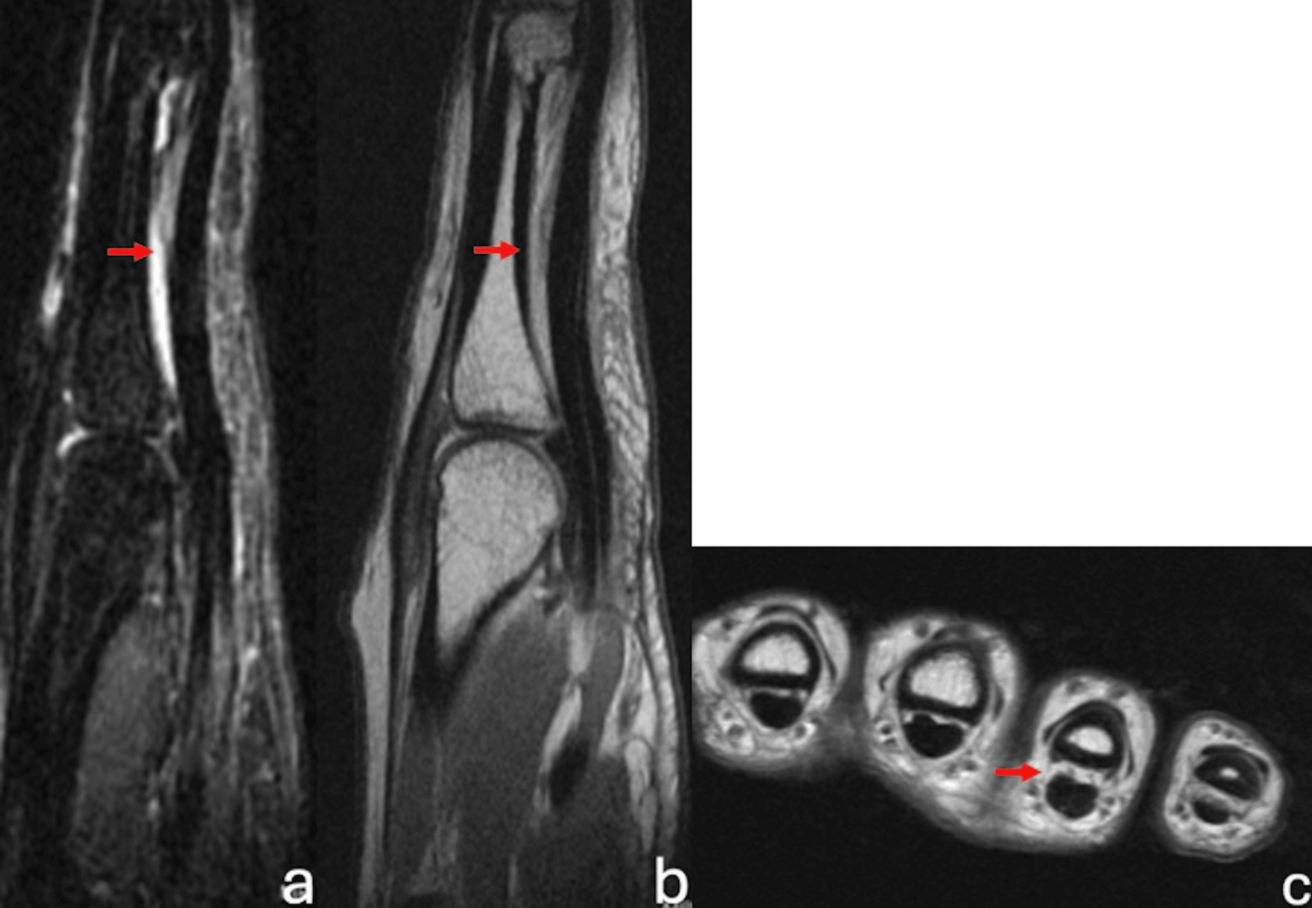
MRI following the initial injury demonstrating an A2 pulley rupture with increased tendon to phalanx distance indicated by the red arrows.
